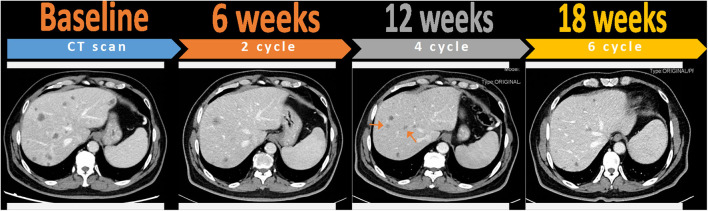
Pseudo-progression of liver metastases after four cycles of toripalimab combined with gemcitabine and nab-paclitaxel, which was confirmed by favorable outcomes at the next assessment of six cycles. Red arrows indicate the appearance of new lesions that were invisible at previous CT evaluation and then disappeared after the continuation of therapy.
